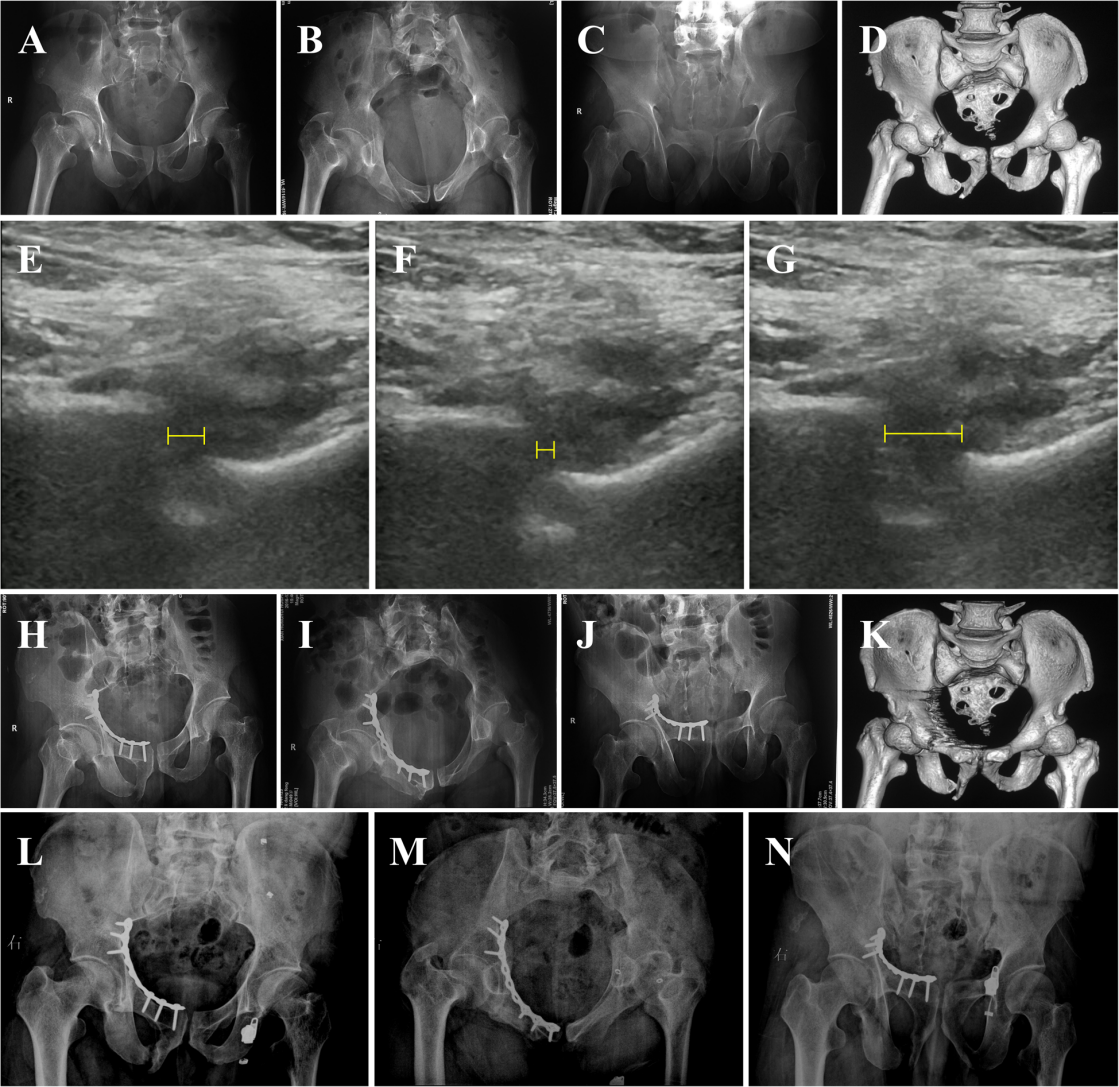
Case 1, 49-y-old male, fell from 3 m height. a-c Preoperative anterior-posterior, inlet and outlet X-rays; d Preoperative CT; e Displacement under ultrasonography when at rest; f Displacement under ultrasonography when compression; g Displacement under ultrasonography when separation. The left-right mobility was 0.72 cm and operation was chosen for the patient. h-j Postoperative anterior-posterior, inlet and outlet X-rays; k Postoperative CT; l-n Postoperative 11 months anterior-posterior, inlet and outlet X-rays. The patient recovered well and Majeed score was 90.
